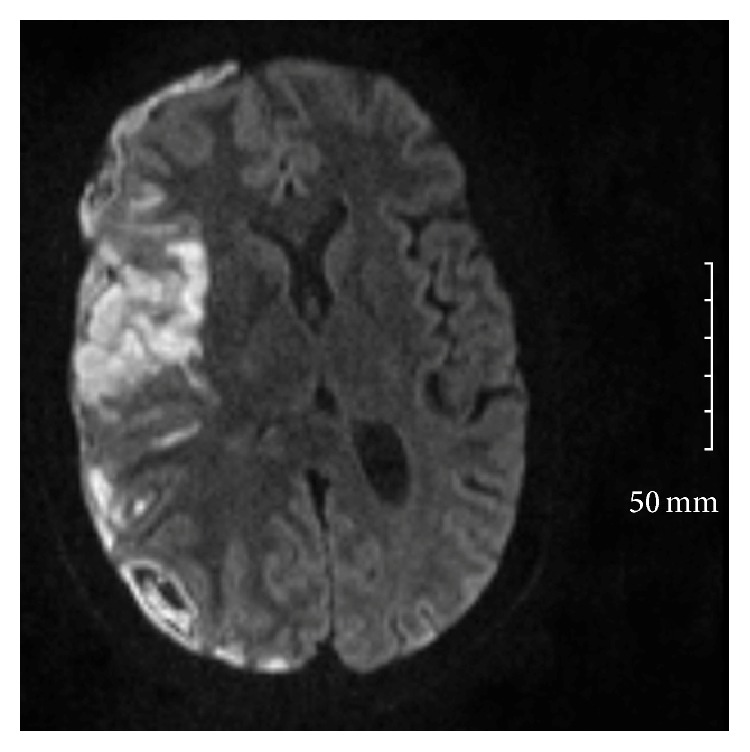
Diffusion Weighted Imaging (DWI) of the same MRI study demonstrating acute right middle cerebral artery territory infarct with loss of flow-related enhancement within the right middle cerebral artery. Per radiologist interpretation, findings may be secondary to associated vasculitis.
